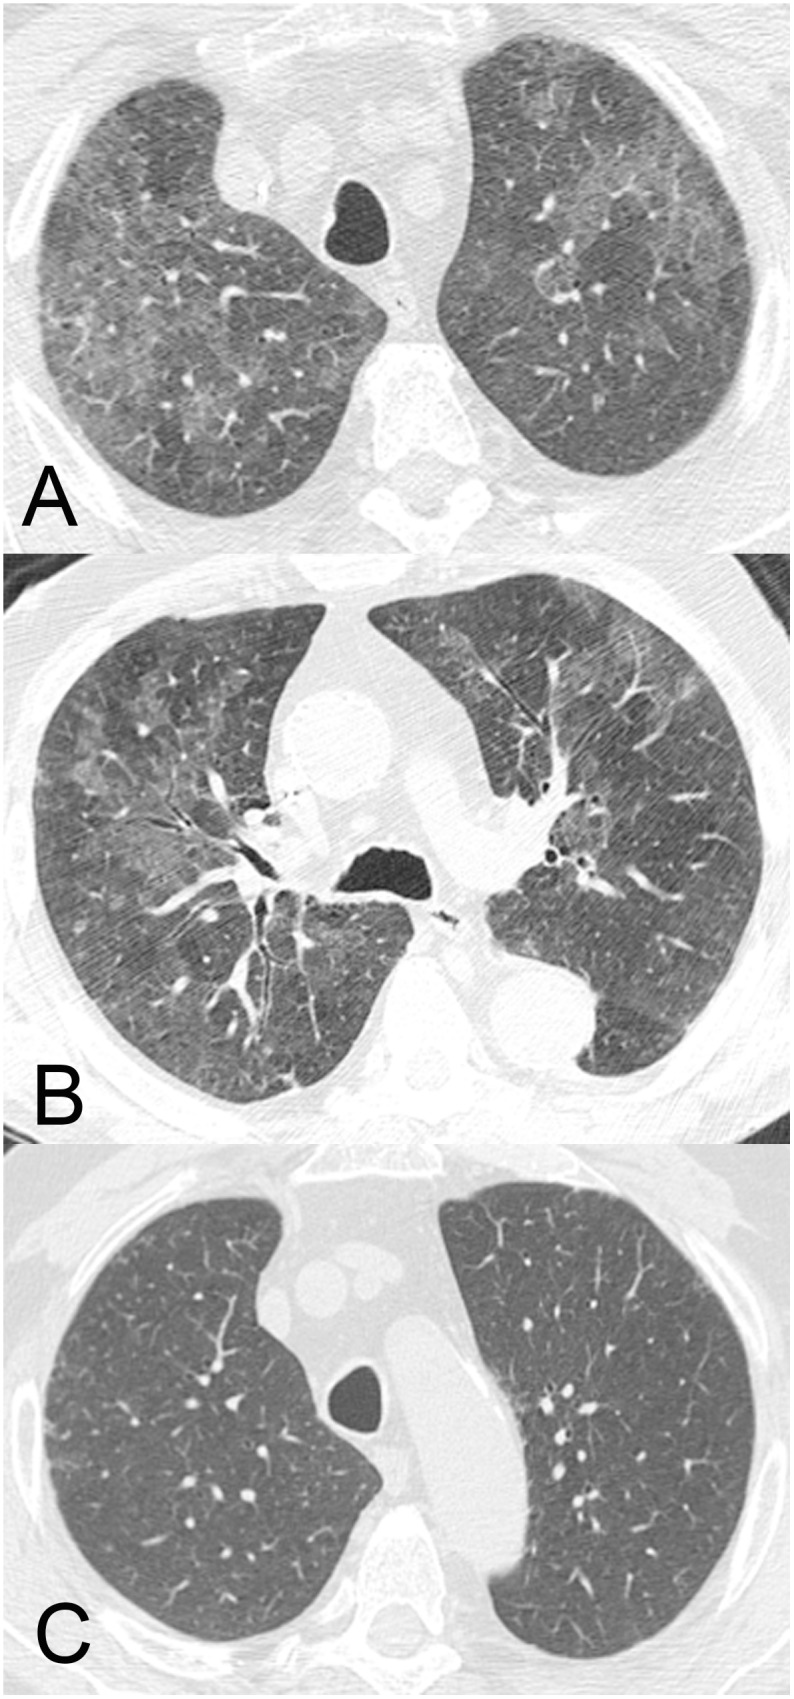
A 78-year-old man with a confirmed diagnosis of dengue. CT images (a, b) show moderate and diffuse ground-glass opacities with no specific distribution in the lungs.
